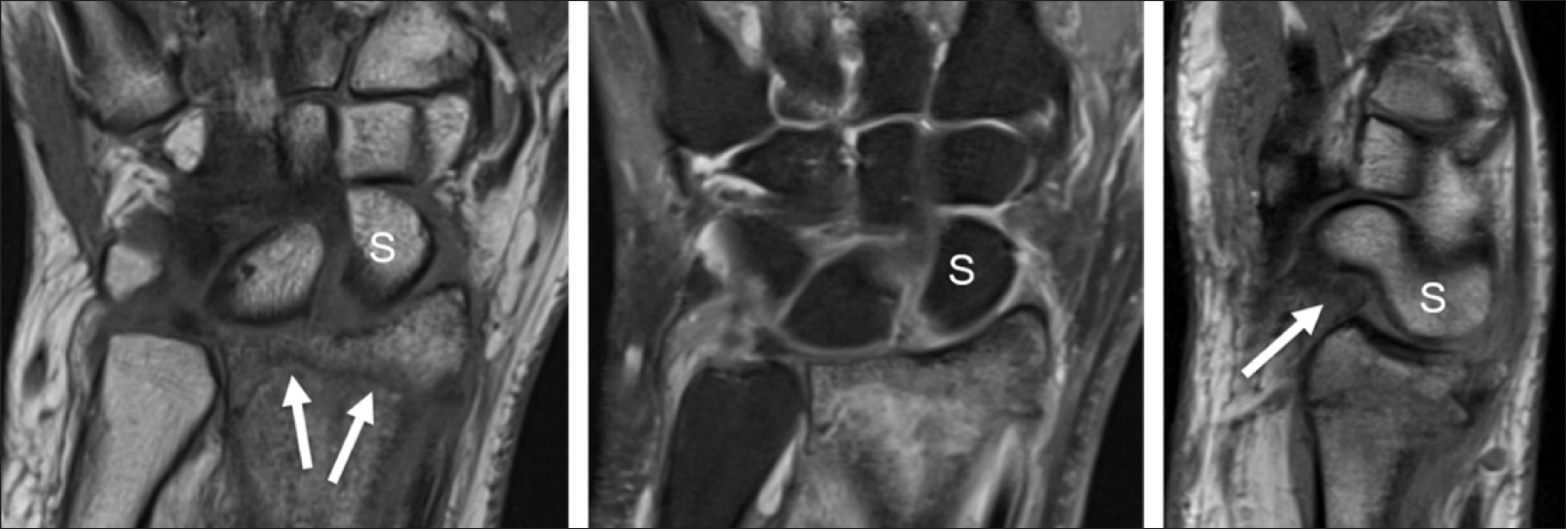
Distal radius fracture with associated intrinsic scapholunate (SL) and extrinsic palmar radiocarpal ligament rupture (RSC, radioscaphocapitate) detected by MRI. T1 coronal slice (a) shows the fracture line (arrows) and the increased SL space. Proton-density fat saturated coronal slice (b) demonstrates high signal surrounding bone marrow edema and increased signal of the SL space. Sagittal proton-density slice (c) detects a dorsal avulsion of the distal radius and a torn palmar RSC ligament (arrow) with rotatory subluxation of the scaphoid (S).
